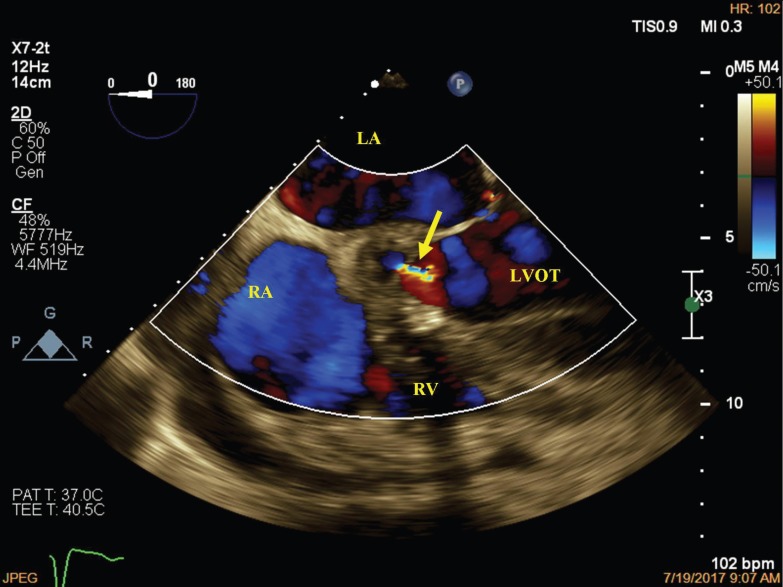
Transesophageal echocardiography in the modified short-axis of the aortic valve view, demonstrating an LVOT-to-RA connection with a systolic shunt (arrow).
